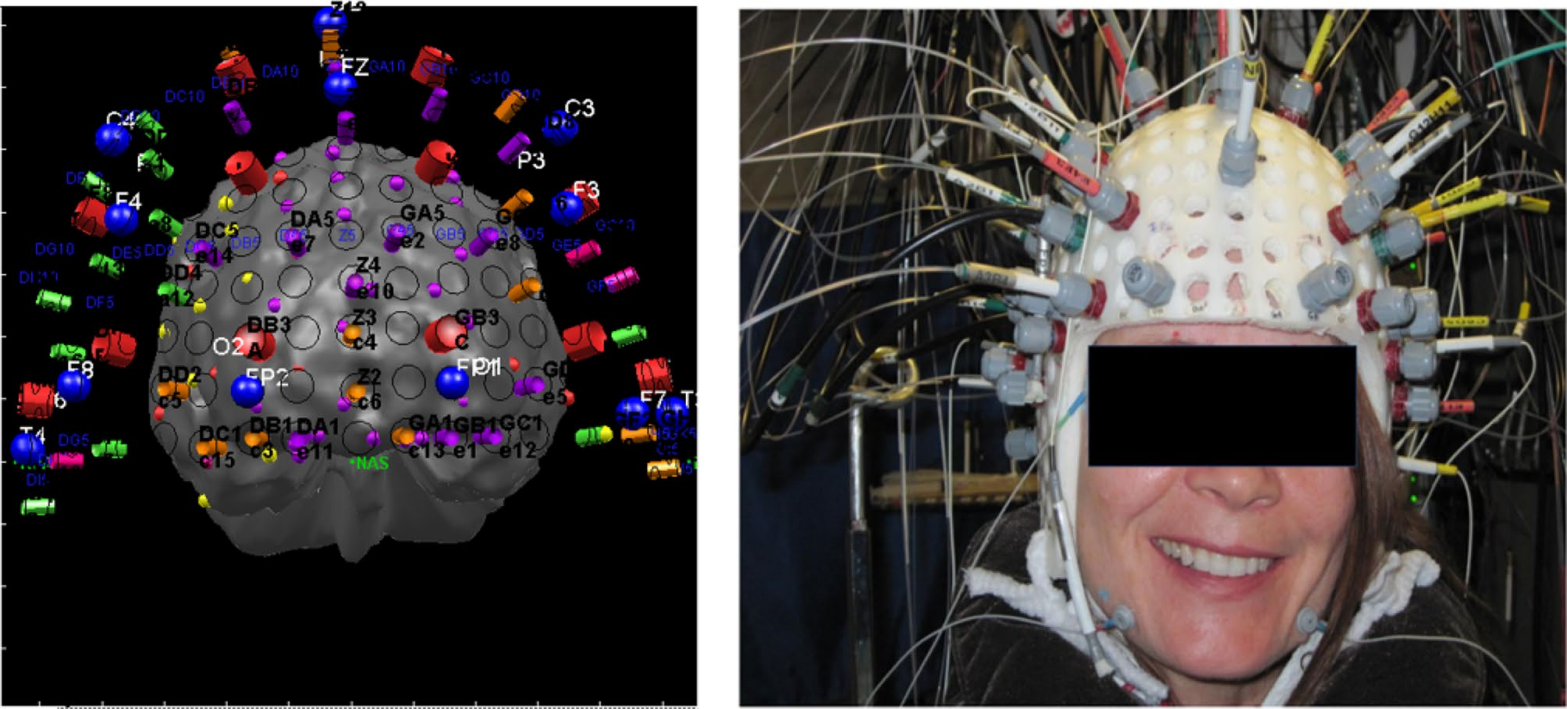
EEG and fNIRS channel-configuration and custom-made multimodal EEG-fNIRS helmets used for EEG and fNIRS data acquisition. Helmets of different sizes and shapes to fit patients’ head comfortably were made from plastic and polyvinyl chloride making them rigid and light. The EEG-fNIRS configuration allows for full head coverage and follows the 10–20 EEG placement system. The fNIRS channel configuration, as well as the EEG (which are in blue dots), are superimposed on the patient’s MRI. We used a 3D camera and stereotaxic system (Frameless 39 from Rogue research) to determine the 3-D coordinates of the optodes relative to the patient’s anatomical MRI
